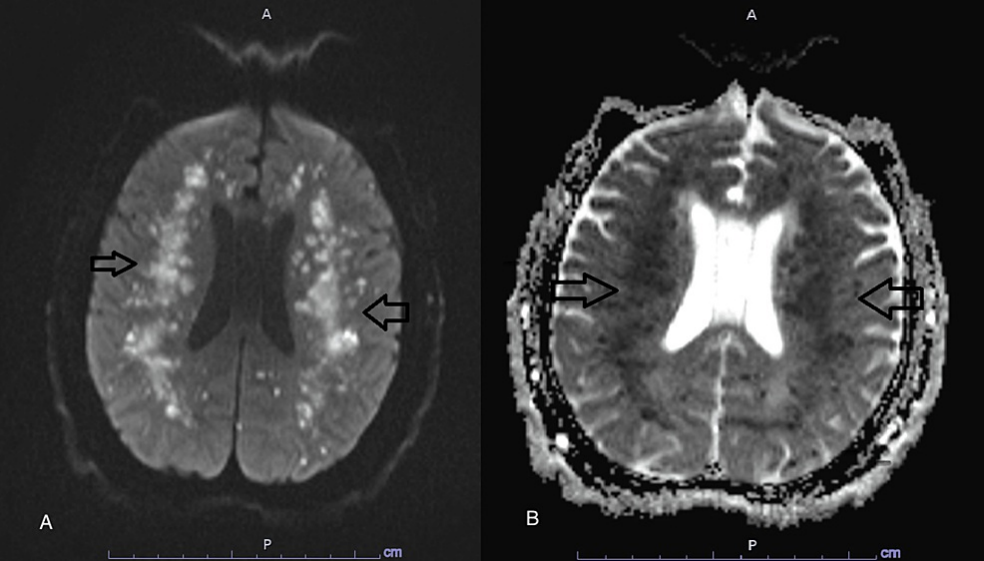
Diffusion trace and diffusion-ADC MRI A is a diffusion trace MRI showing the extensive white matter strokes (arrows), and B is a diffusion-apparent diffusion coefficient (ADC) window showing corresponding defects (arrows)
